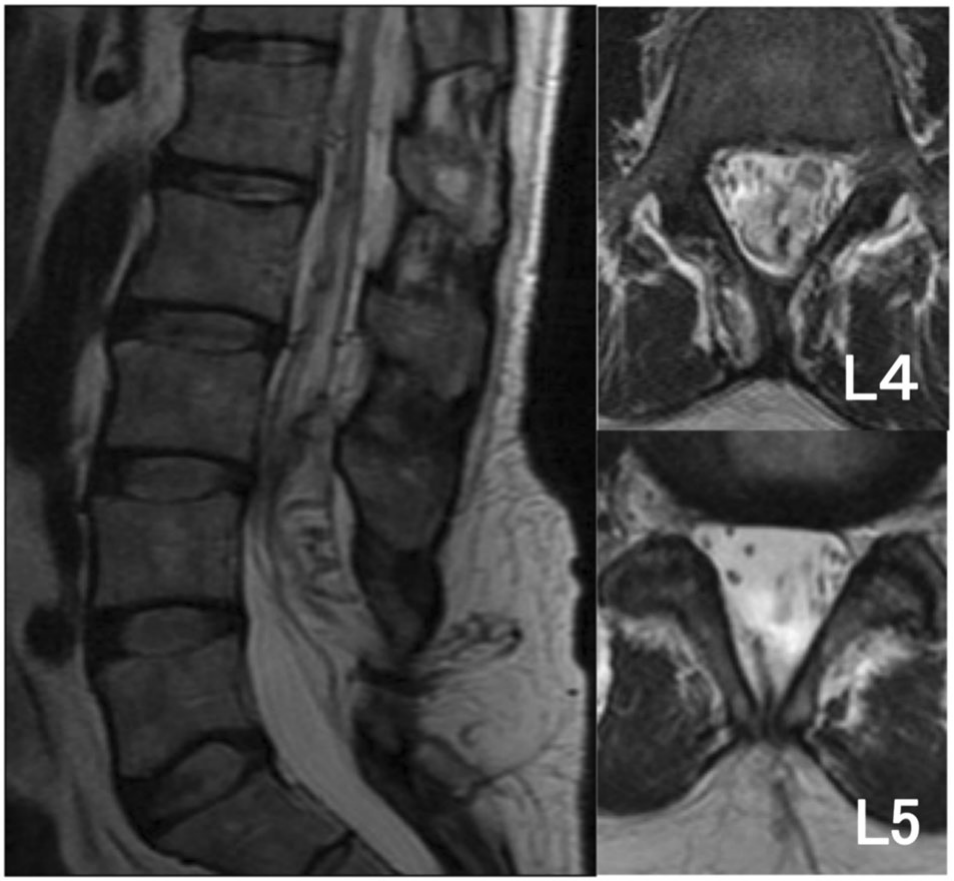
Case 1. T2-weighted MRI scan showing a spinal lipoma at the L5 level with a flow void dorsal to the spinal cord
